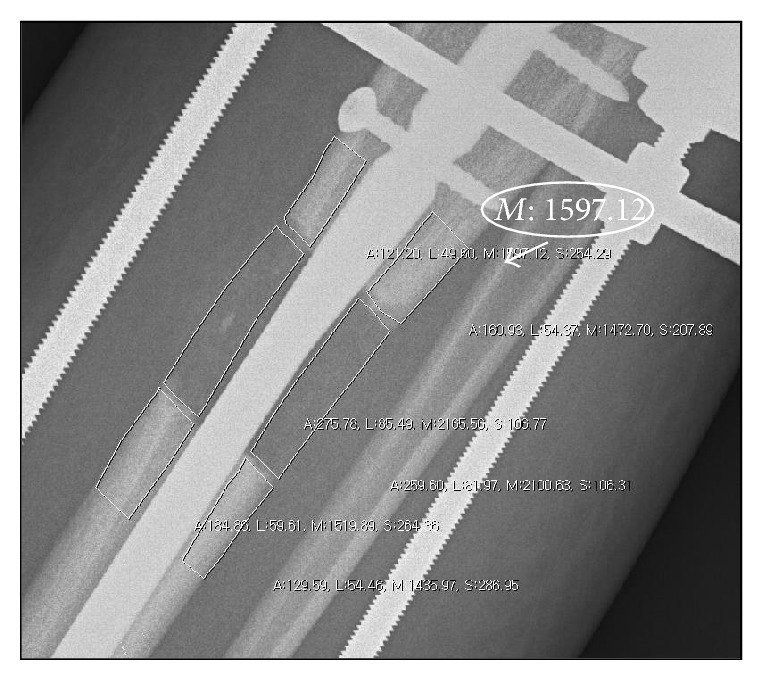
The radiograph shows how to measure the pixel values on a digital radiograph. The different cortical segments of callus and the proximal and distal segments were measured with use of the free region of interest methods of StarPACS PiView Star 5.0.6.1 software (Infinitt Co., Ltd., Seoul, Korea). White circled “*M*” value was the pixel value of the region of interest drawn.
